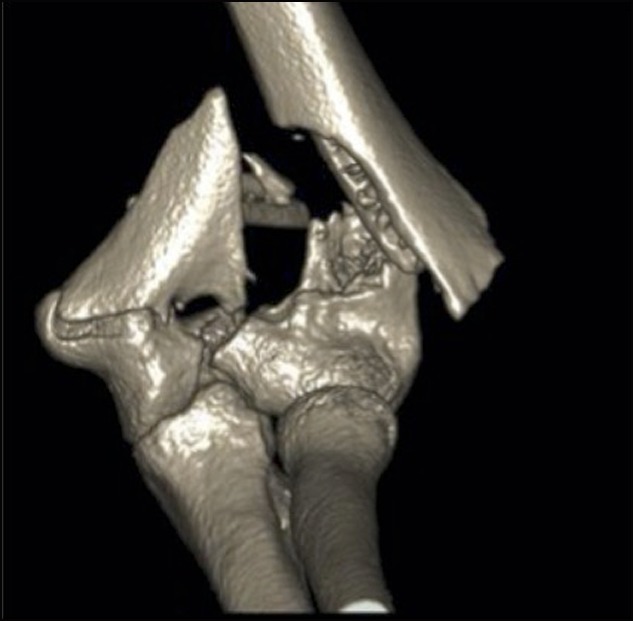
Three-dimensional CT scan in a 15-year-old boy with a T-type distal humerus fracture, to aid with preoperative planning.
